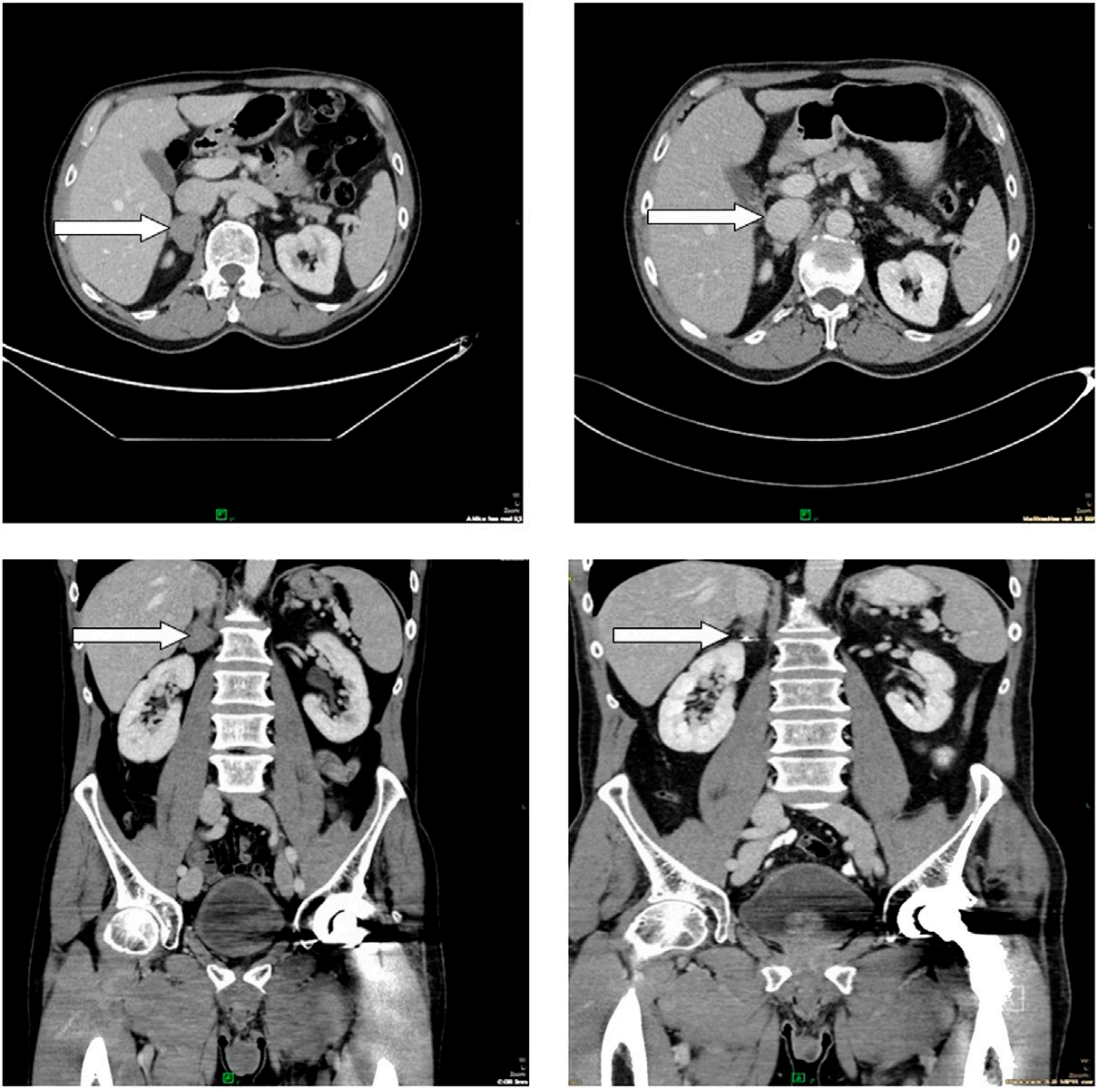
Follow-up CT scans show partial regression in the adrenal gland, 6 months after the radiotherapy. The patient received checkpoint inhibitor, as systemic therapy.
